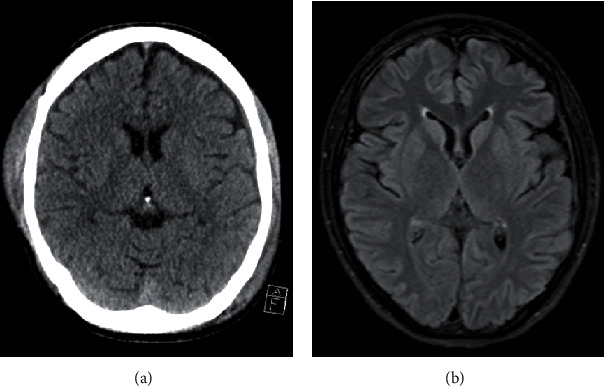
Cranial CT scan (a) and cranial MRI scan (b) revealed evidence of a small right-sided subdural and intracerebral hematoma.
